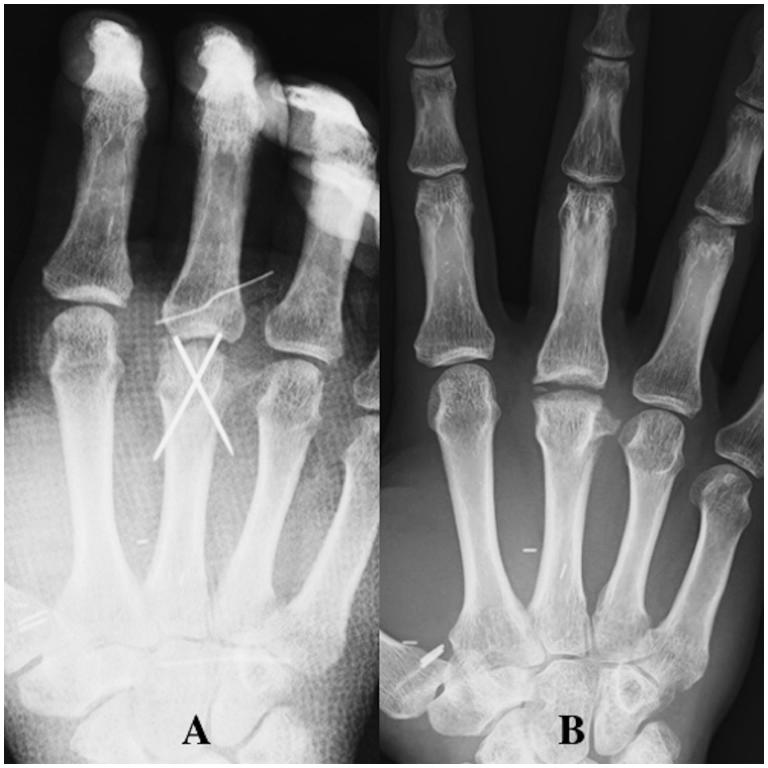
Case 2. (A) The osteochondral bone segment fixed by K-wires. (B) Radiography showed a thick layer of cartilage at the MCP joint without joint space narrowing at final follow up.
